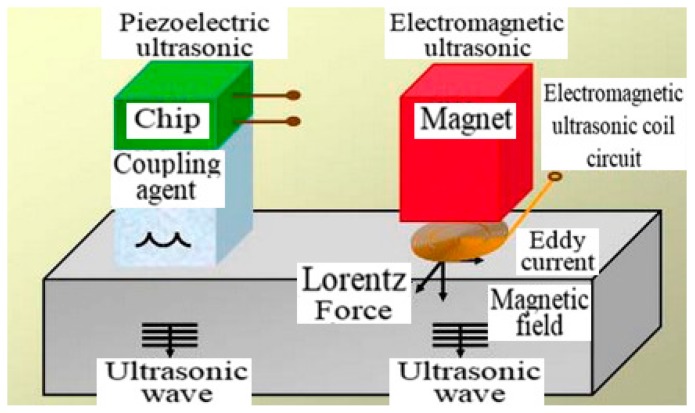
The comparison of electromagnetic and piezoelectric ultrasonic testing.
